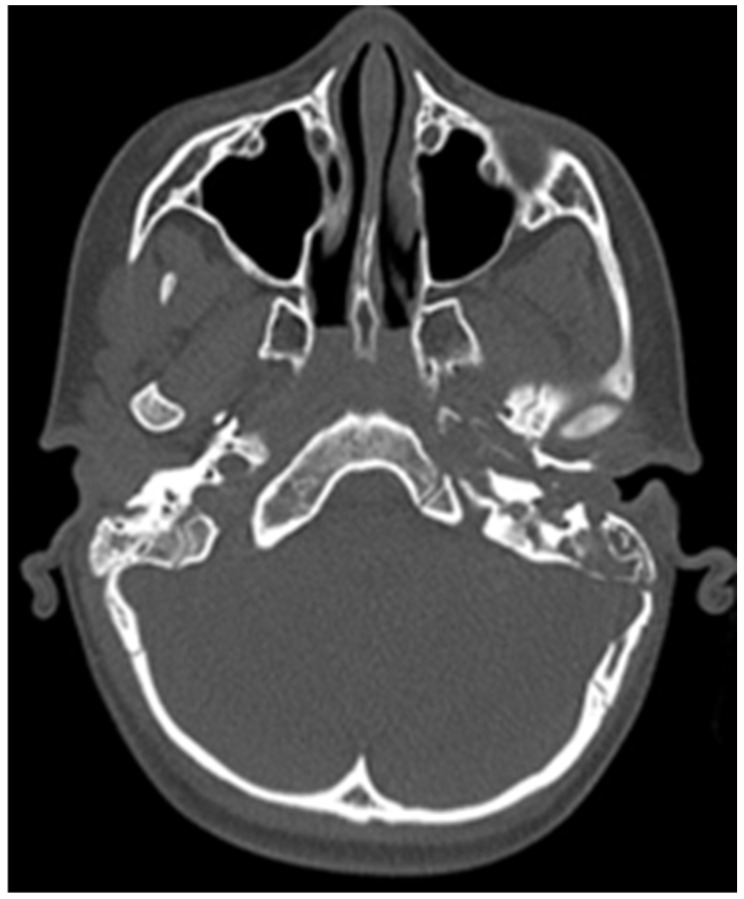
Initial CT of the patient.
